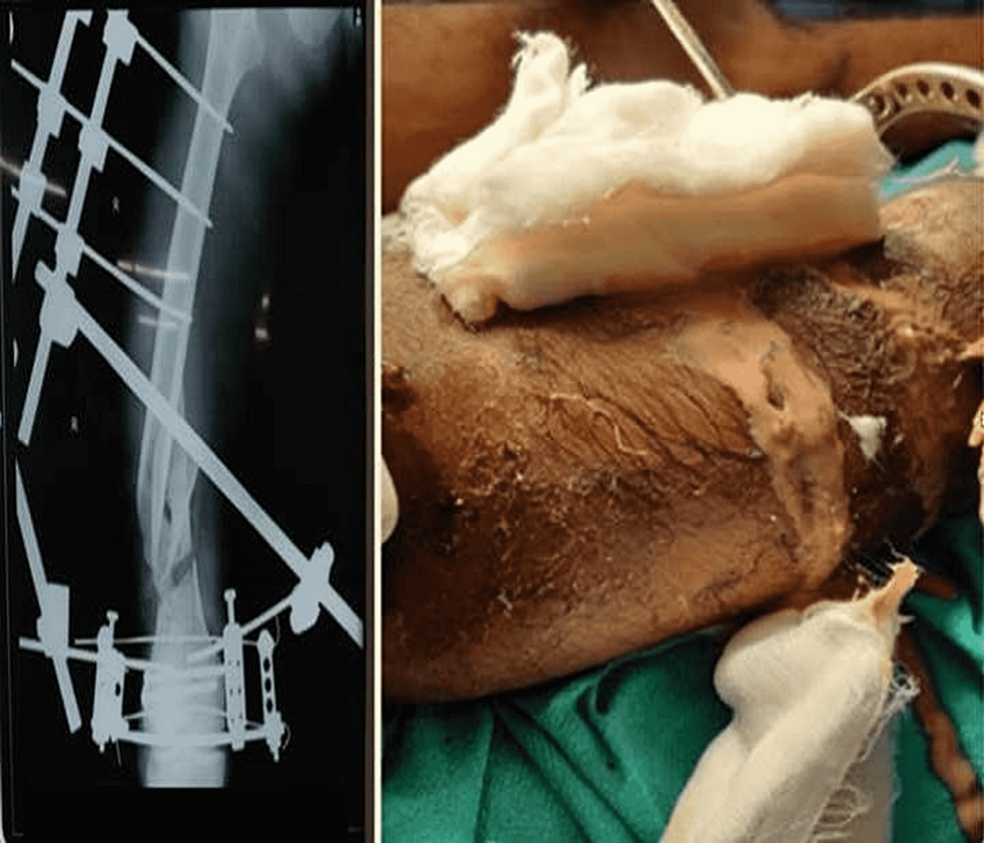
X-ray right femur showing external fixator over distal femur and clinical image of pouring pus after infection
